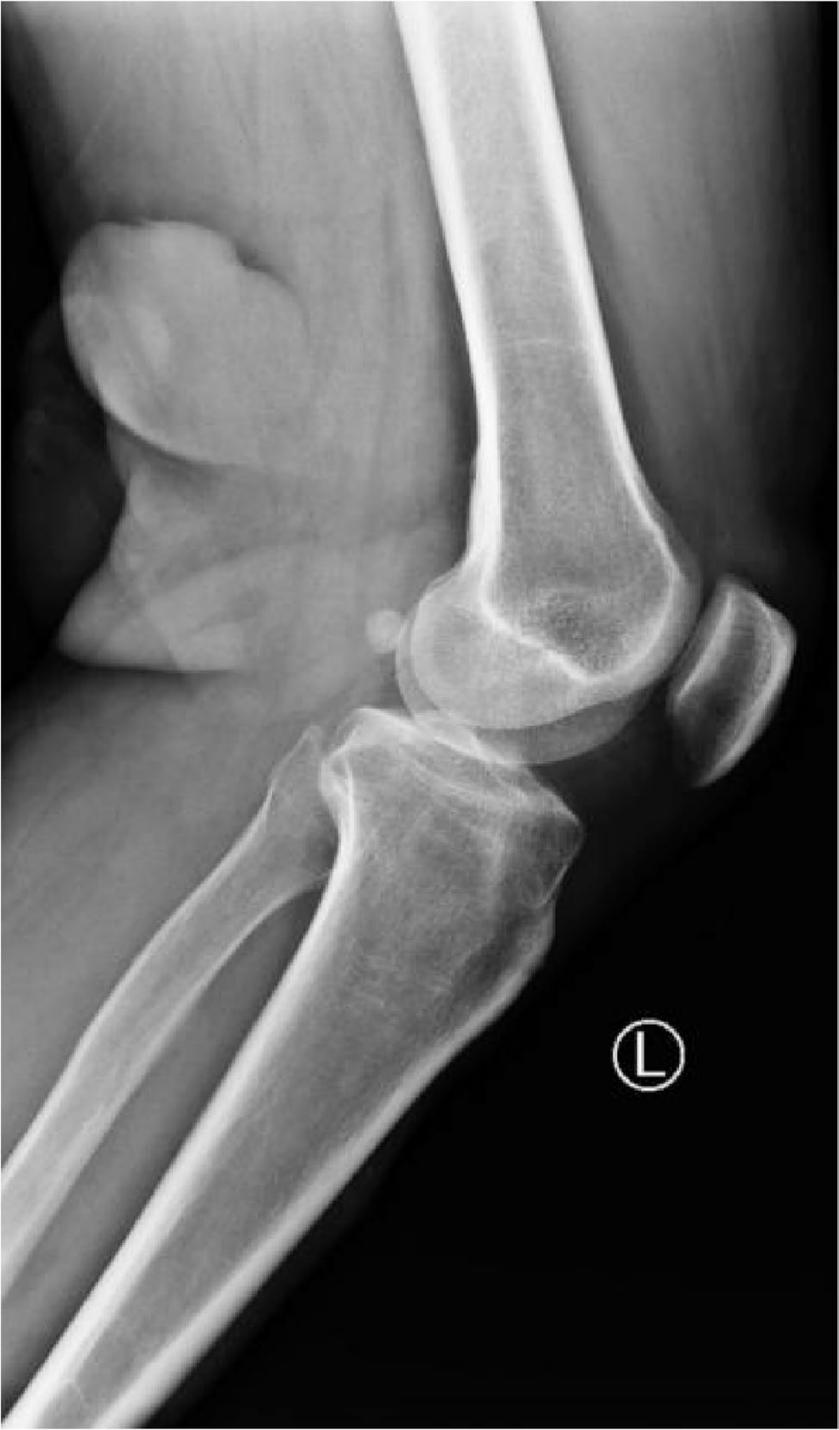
2023.03 X-ray shows a round mass is observed in the left popliteal fossa, with clear boundaries and a distinct separation from the femur. (This refers to the lesion mentioned in the past medical history from 1.5 years ago, which has since been treated with surgery and radiotherapy.)
